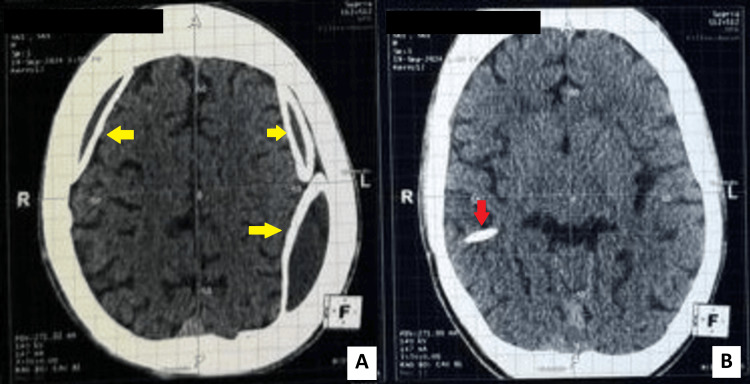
Preoperative axial non-contrast CT of the head. (A) CT scan of the head showing bilateral dense subdural laminar calcified collections encasing the convexity (yellow arrows). (B) CT scan of the head showing the VP shunt catheter projection toward the right lateral ventricle (red arrow) with a collapsed aspect of the lateral ventricle related to hyperdrainage. VP: ventriculoperitoneal
